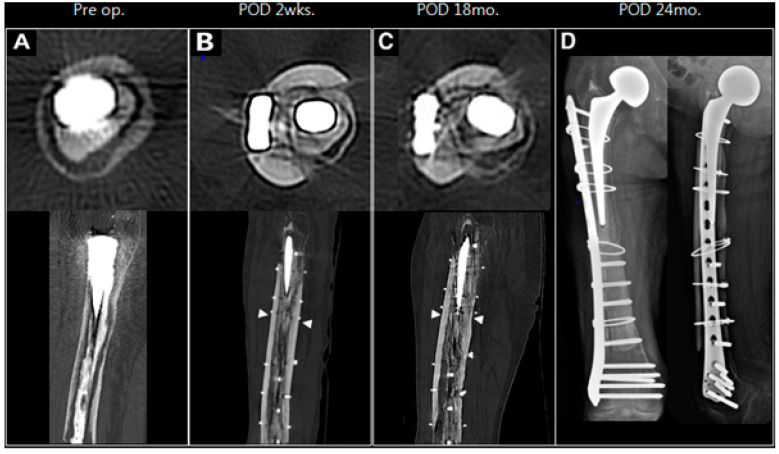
The axial and sagittal section of the femur showing the secondary stability of the cemented stem. (A) The preoperative CT scan shows a gap between the cortical bone and the cement. (B) Note the thick strut allografts placed anterior and posterior to the fractured femur, providing stability to the intramedullary cement (arrowhead). (C) Bone integration is confirmed, and stem stability was maintained after 18 months. (D) The anteroposterior and lateral views of the femur at 24 months following the operation show the unchanged position of the femoral stem as compared to the immediate postoperative status.
